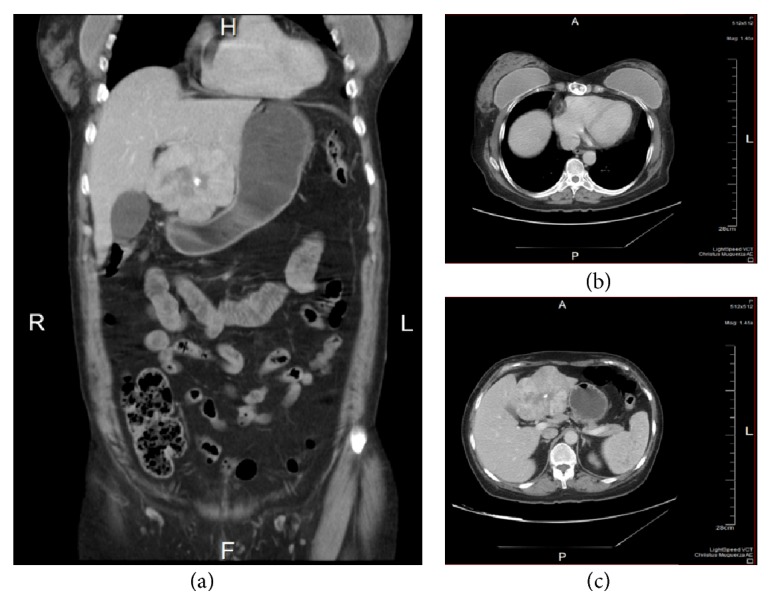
Images from the abdominal CT. (a) and (b) show the 8 × 8 × 7.3 cm tumor located in segment IV of the left lobe of the liver that laterally displaces the gallbladder. The lesion has defined lobular borders that show a heterogeneous peripheral areas and a hypodense center with calcification. (c) shows changes in the sternum with presence of surgical material.
